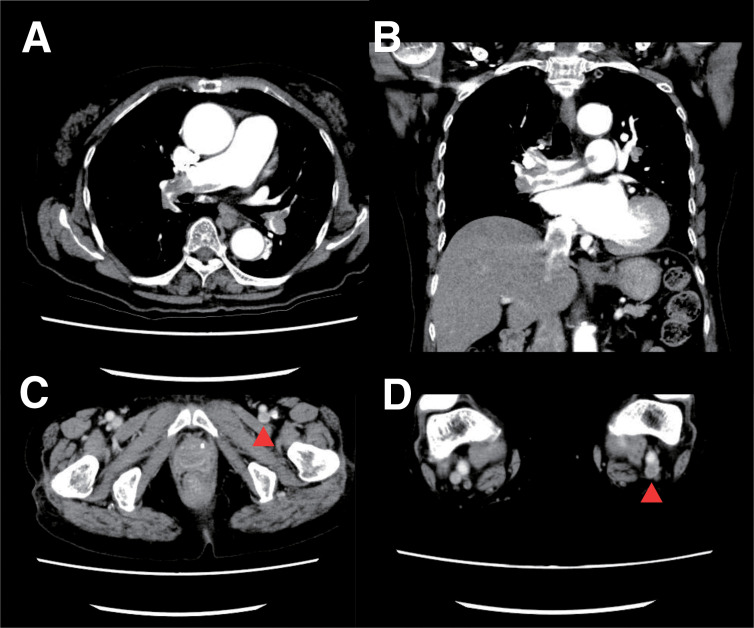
Contrast-enhanced computed tomography performed 14 days after CAC. (A) Massive thrombi are present in the bilateral pulmonary arteries (short-axis view). (B) Massive thrombi are present in the bilateral pulmonary arteries (long-axis view). (C) Deep vein thrombosis was detected in the left common femoral vein (indicated by the red arrowhead). (D) Thrombi were detected in the left popliteal vein (indicated by the red arrowhead). Superficial vein thrombi were also identified in bilateral small saphenous veins (not shown in this slice). CAC: cyanoacrylate closure
